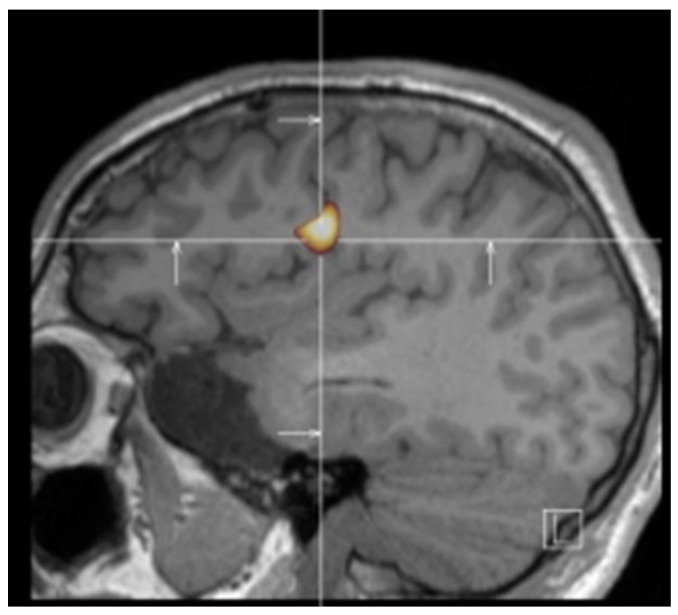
Cerebral MRI (sagittal view) and ictal/interictal technetium-99m HMPAO (99mTc-HMPAO) SPECT subtraction using BRASS analysis program in a patient with ecstatic epilepsy. It shows an increased blood flow maximal at the junction of the right dorsal mid-insula and central operculum. Adapted from a Figure published in Cortex 49 (2013), F. Picard, State of belief, subjective certainty, and bliss as a product of cortical dysfunction, page 2495, Reproduced with permission from Elsevier.
